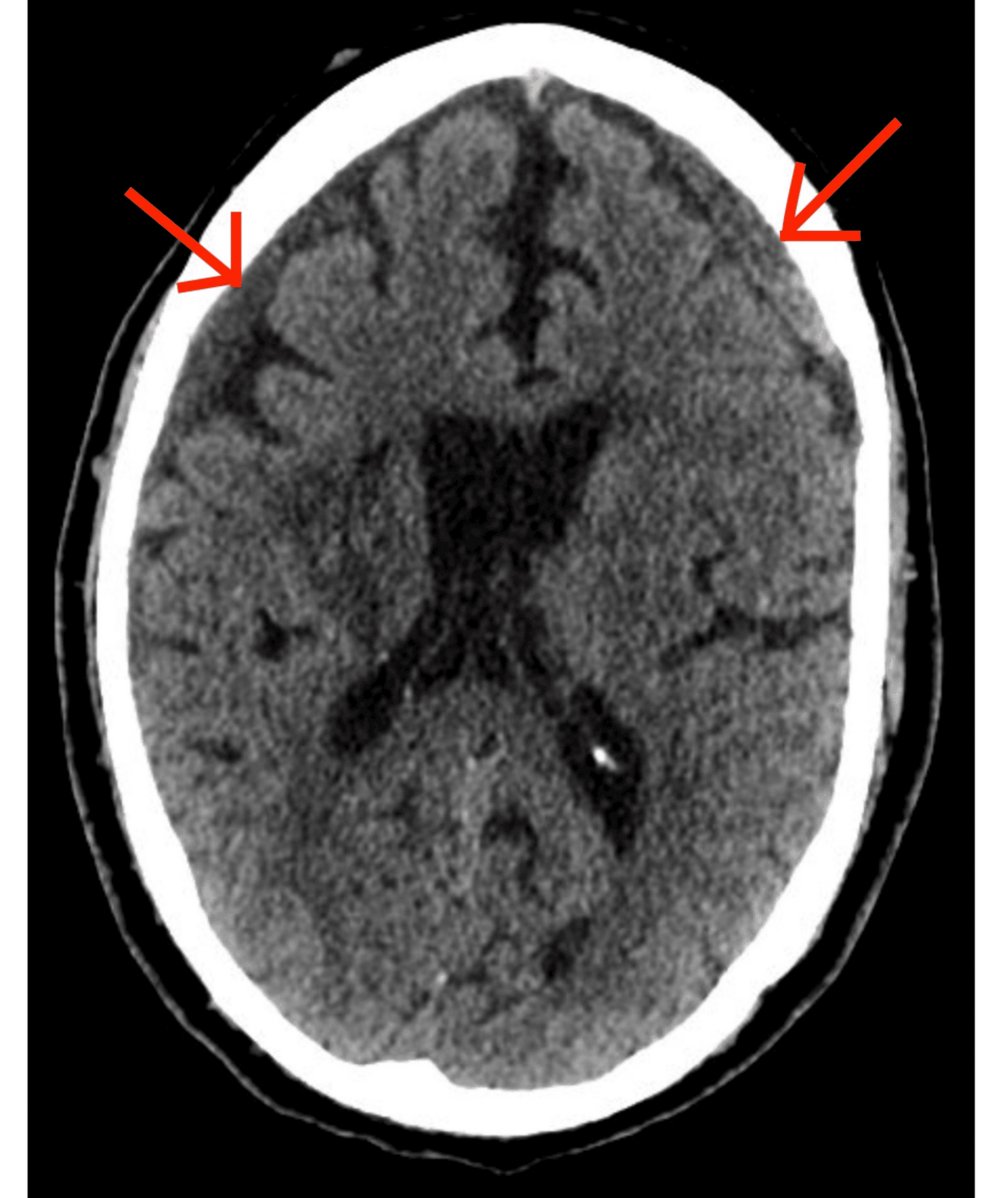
Non-contrast CT scan (axial) - brain - week 14. Bilateral subdural hematomas (SDH), with a stable left SDH and an expanding right SDH, on a background of resolving right intraparenchymal hemorrhage (IPH). Varying intensities are seen in the left-sided SDH, ranging from hypodense to a few areas of hyperdensity.
